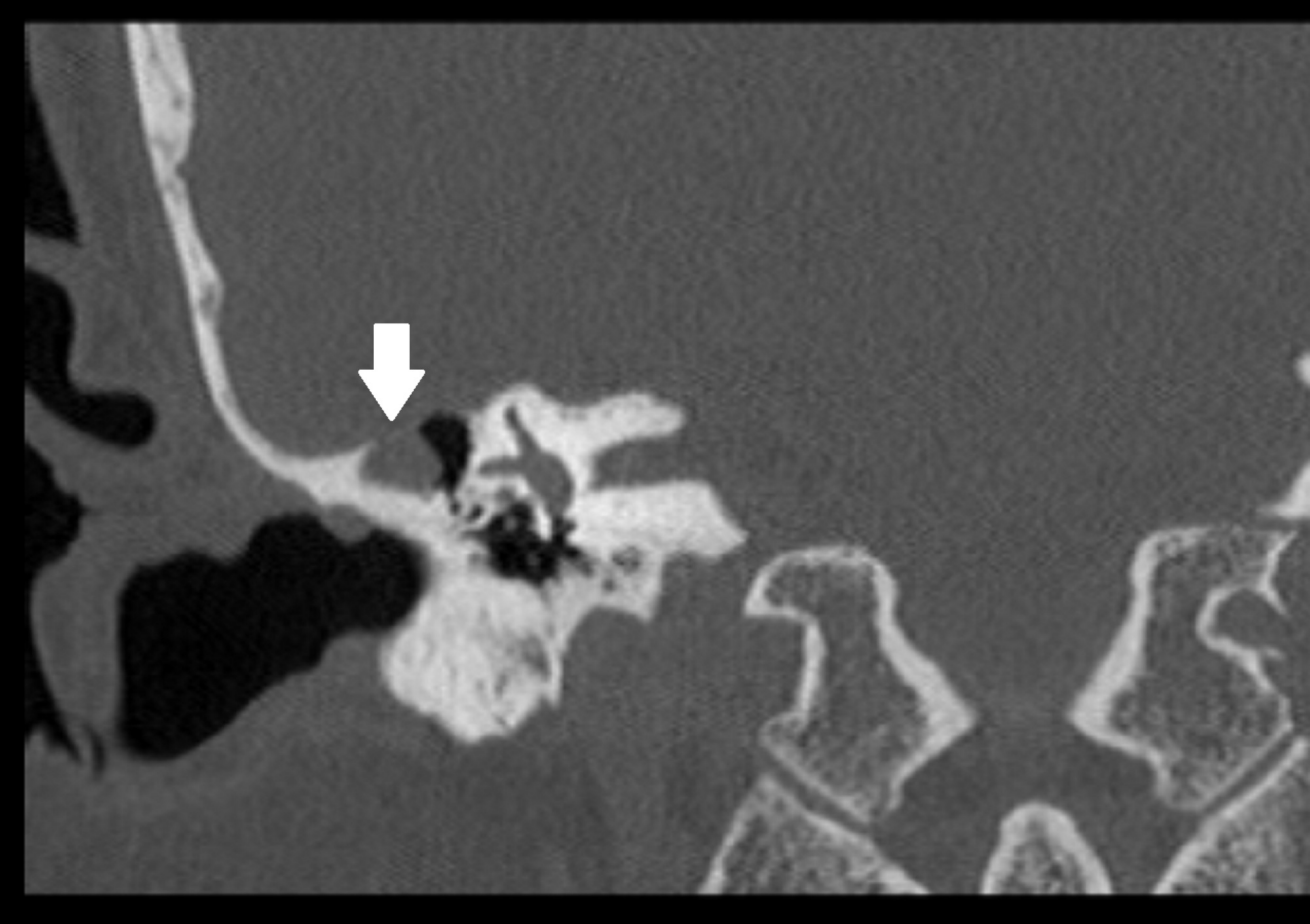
High-resolution CT scan highlighting the temporal bone defect and the associated encephalocele CT: computed tomography
